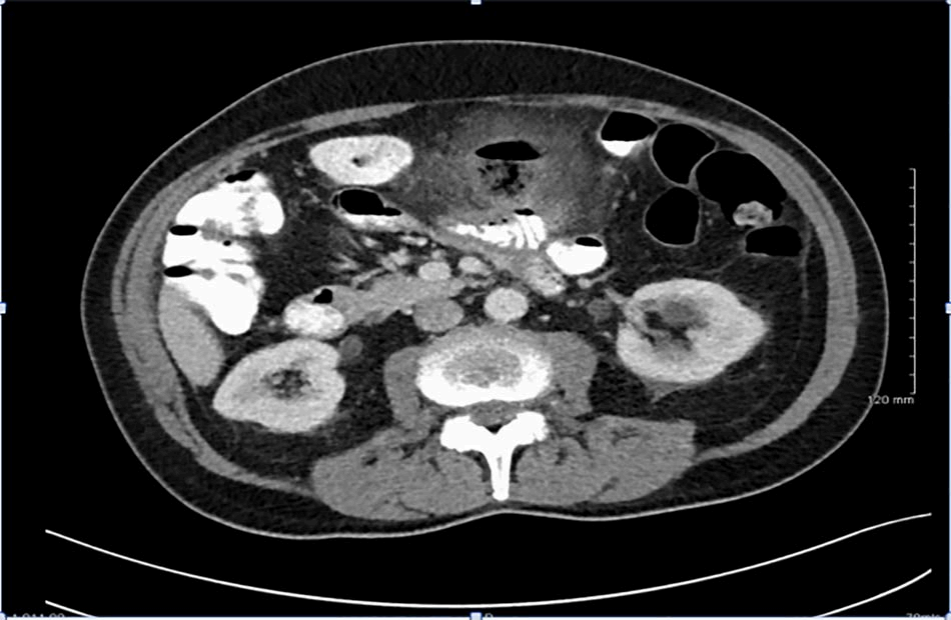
CT findings demonstrating large inflammatory mass related to perforated jejunal diverticulitis.
